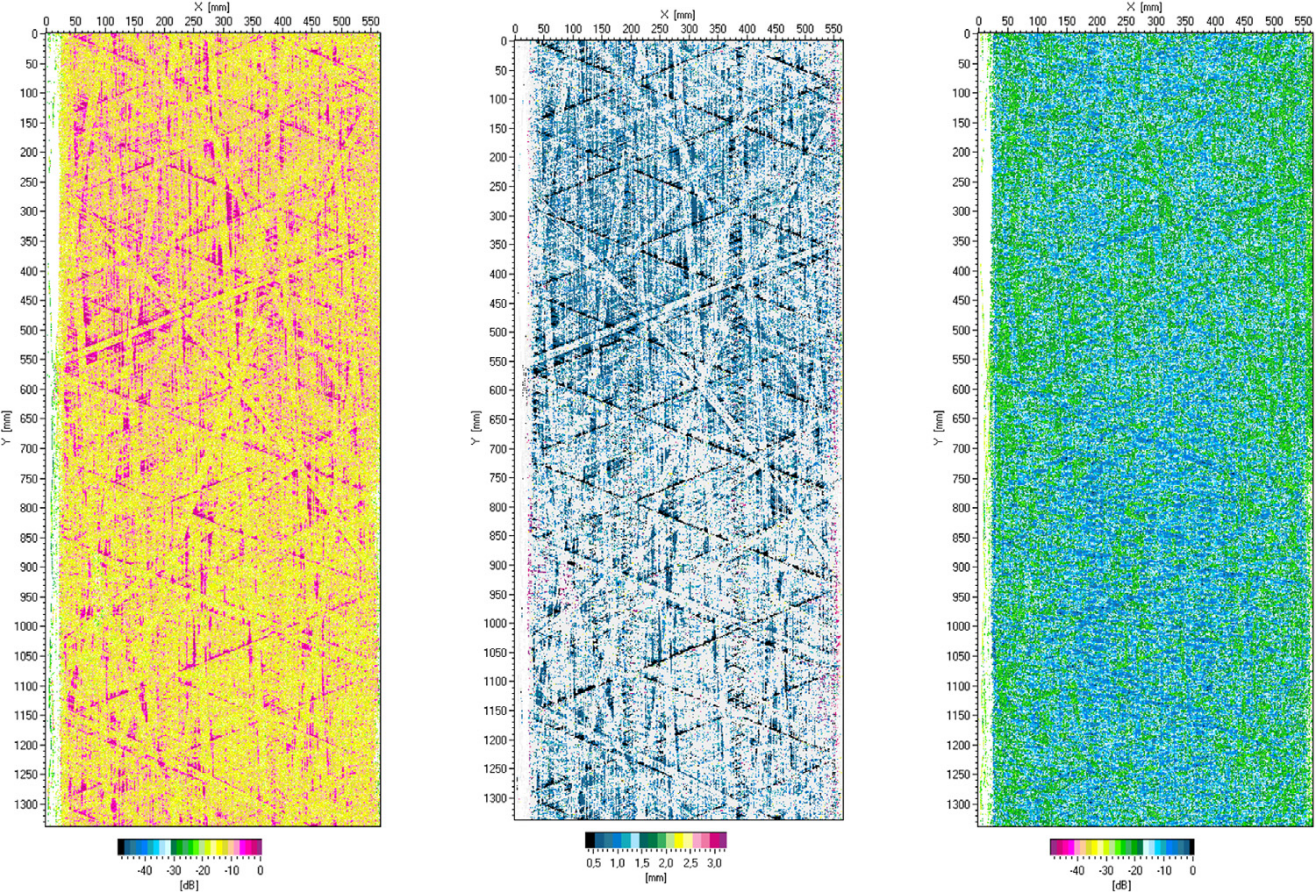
Ultrasonic scan results for cylindrical part of tested vessel SN03: C-Scan of the flaw echo (left), D-Scan providing flaw depth (centre) and C-Scan of the back-wall echo (right).
